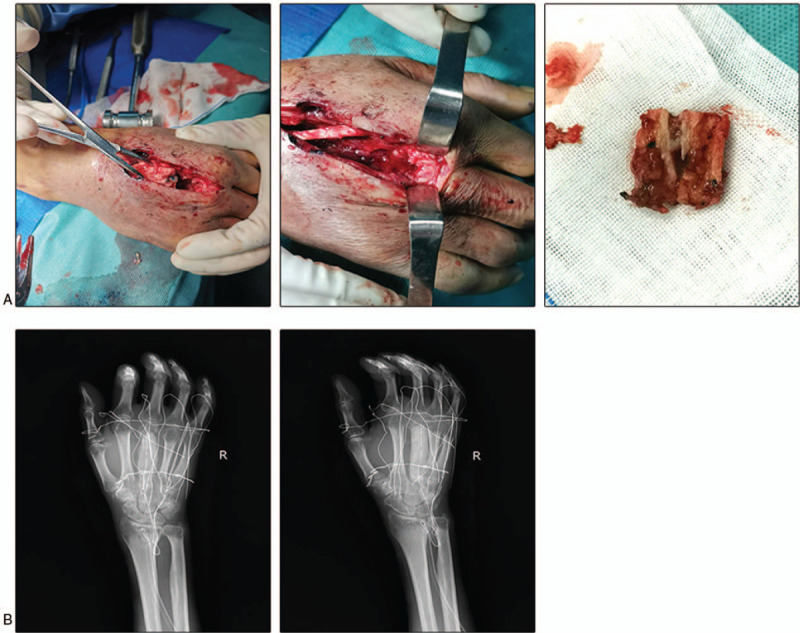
(A) Images during operation. The lesions around the metacarpal bones are wrapped with cheese-like tissue, combined with severe bone destruction. (B) X-ray image after surgery.
